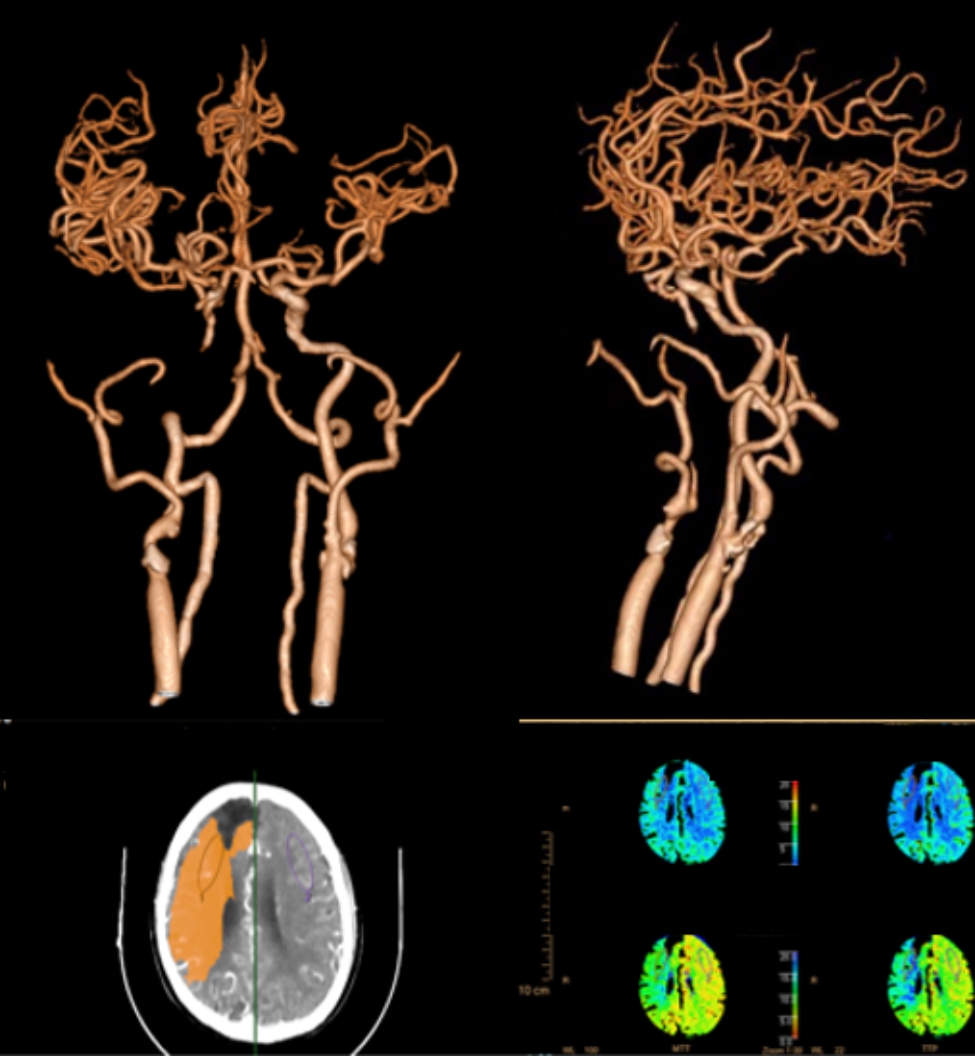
CTA and CTP three days after admission
